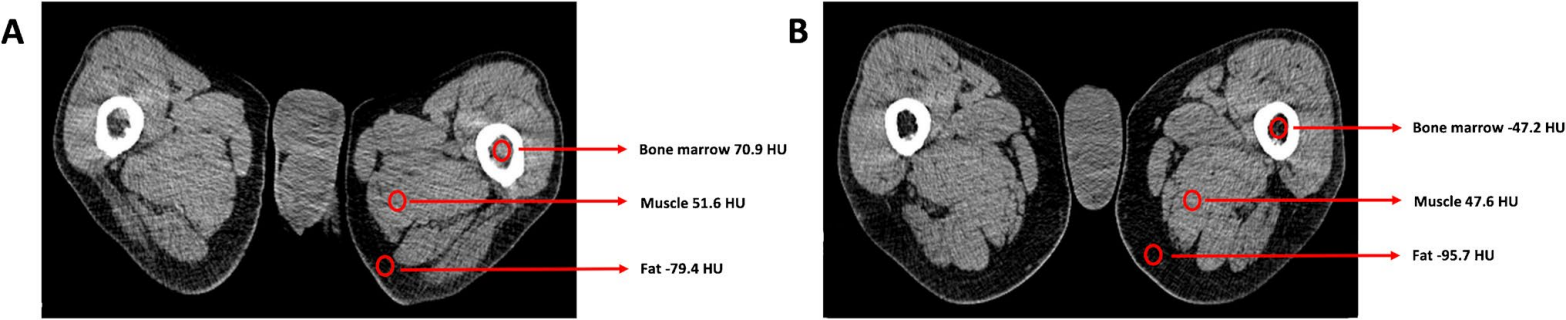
A previously untreated male MM patient with, R-ISS stage II, and a BM plasma cell infiltration rate of 76%. The patient received induction therapy with isatuximab in combination with lenalidomide, bortezomib, and dexamethasone. Following induction therapy, the patient achieved a very good partial response (VGPR) according to IMWG criteria, while MRD remained positive. Shown are representative axial CT images at the level of the femora and results from the manual CT-based quantification of BM infiltration in the appendicular skeleton before (A) and after (B) induction treatment. The highest mean BM CTv decreased from 32.7 HU to -26.6 HU, reflecting a treatment-associated reduction in BM density
